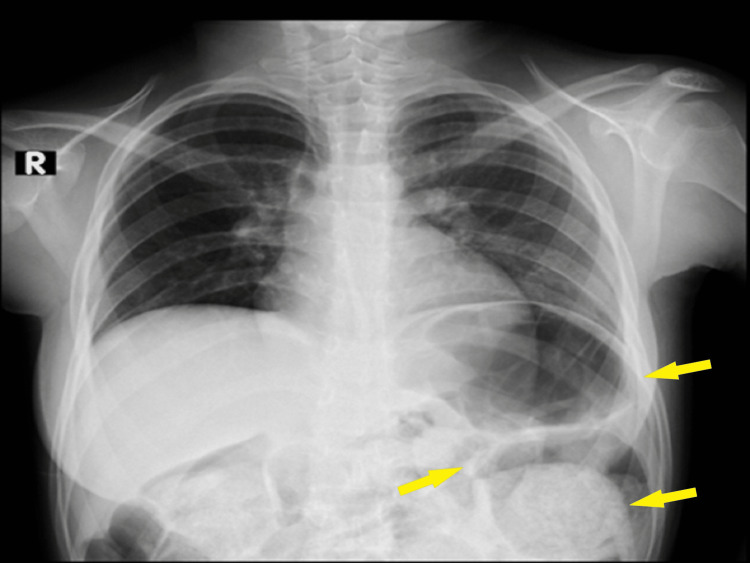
The patient's chest X-ray Yellow arrows show a dilated bowel pushing the diaphragm superiorly, creating a mass effect on the left side.
